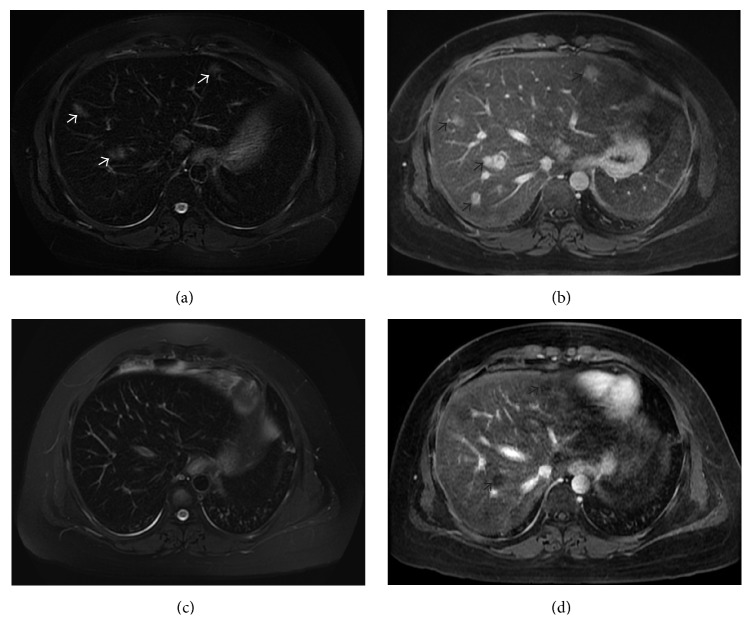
(a, b) Baseline MRI. (a) Axial T2-weighted fat saturated (T2W-FS) image shows faintly hyperintense lesions in the liver (white arrows). The liver parenchyma is hypointense and shows reduced signal in keeping with iron loading. (b) Axial delayed postcontrast T1W images show persistent delayed enhancement in the lesions (black arrows). (c, d) MRI after treatment. (c) Axial T2W-FS image shows that the lesions are isointense to the rest of the liver parenchyma which is hypointense (due to iron loading). The lesions are no longer hyperintense on the T2W-FS image. (d) Axial delayed postcontrast T1W images show that the lesions no longer show any appreciable enhancement.
